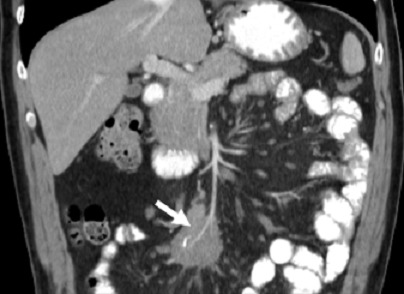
Coronal CT scan with contrast, demonstrating vascular encasement within the mesenteric lesion
